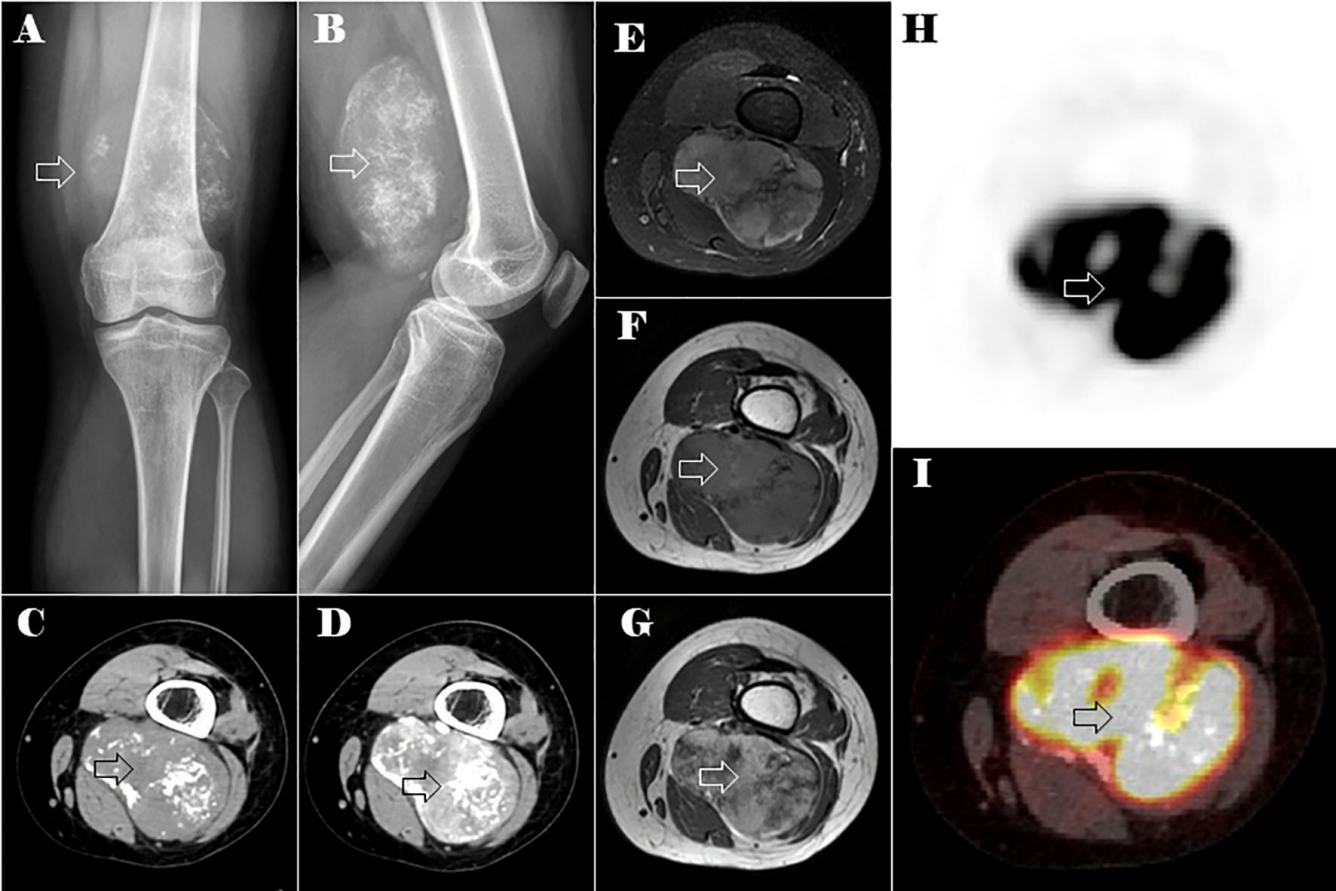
Plain X-ray, CT, MRI, and PET-CT images of the lesion in the left distal thigh (the lesion is indicated by the hollow arrow). Anteroposterior (A) and lateral (B) plain x-ray showed an oval soft tissue mass with spotty calcifications. Axial CT of soft tissue window images in the unenhanced (C) and enhanced (D) phases. Axial MRI images of the tumor mass on non-contrast T1-weighted (E), T2-weighted fat-suppression (F), and T1 enhanced (G) images. Axial PET-CT images of the lesions in the left distal thigh (H, I).
